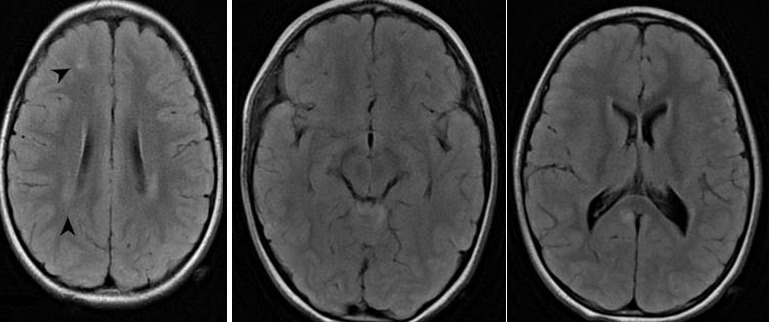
Sagittal T2 FLAIR High signal changes indicating diffuse axonal injuries in corpus callosum, cerebellar, and basal ganglia of the right hemisphere
